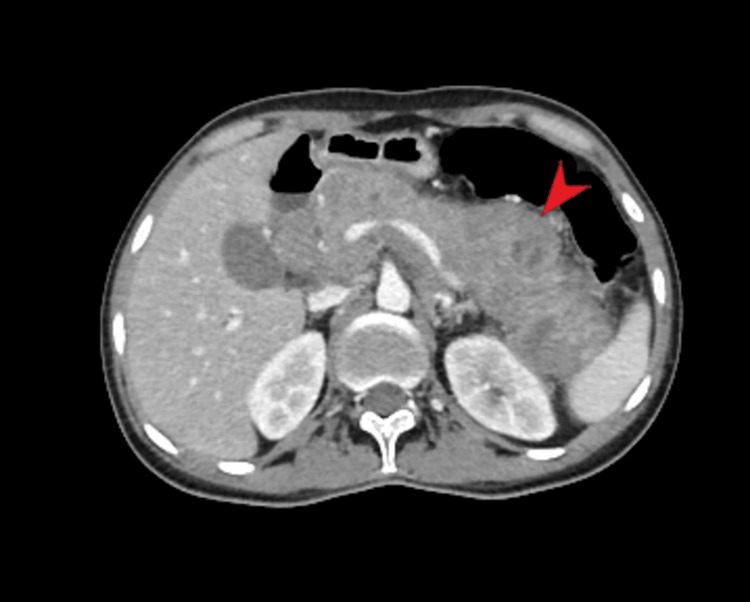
Selected axial post-contrast contrast-enhanced CT (CECT) section showing diffusely swollen heterogeneous relatively hypoenhancing pancreas annotated by the red arrow.
